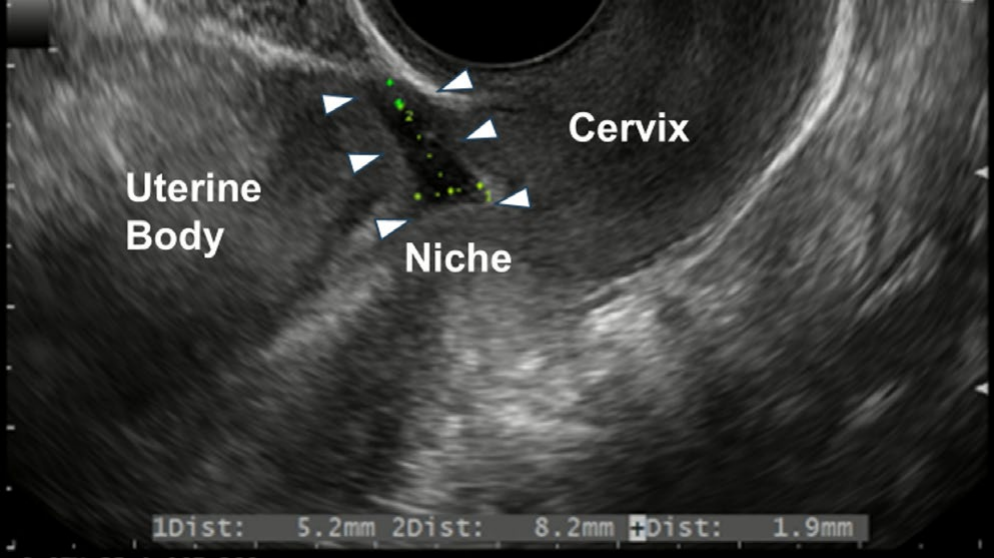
Imaging findings of a cesarean scar niche on transvaginal ultrasonography. Transvaginal ultrasonography identified a cesarean scar niche interposed between the uterine cervix and the uterine body, with dimensions of 5.2 mm in length and 8.2 mm in depth. The residual myometrial thickness (RMT) at the thinnest point measured 1.9 mm. These imaging findings were indicative of a cesarean scar disorder.
